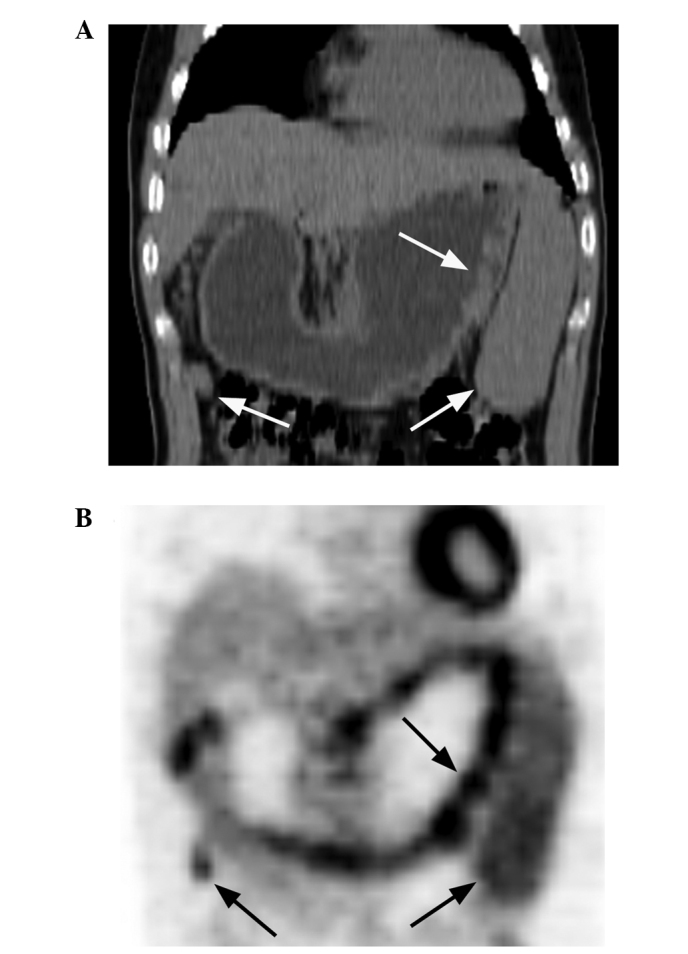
A 48-year-old male with gastric mucosa-associated lymphoid tissue lymphoma. (A) In the coronal computed tomography image, a diffuse thickened gastric wall with multiple nodules can be observed in the fundus, body and antrum of the stomach. Enlarged perigastric lymph nodes and spleen can are also apparent in this image. (B) In the coronal positron emission tomography image, a string-of-beads pattern of high FDG uptake (maximal standard uptake value, 7.9) can be confirmed in the corresponding gastric regions. Perigastric lymph nodes and spleen with increased FDG uptake are also apparent in this image. FDG, ^18^F-fluorodeoxyglucose.
